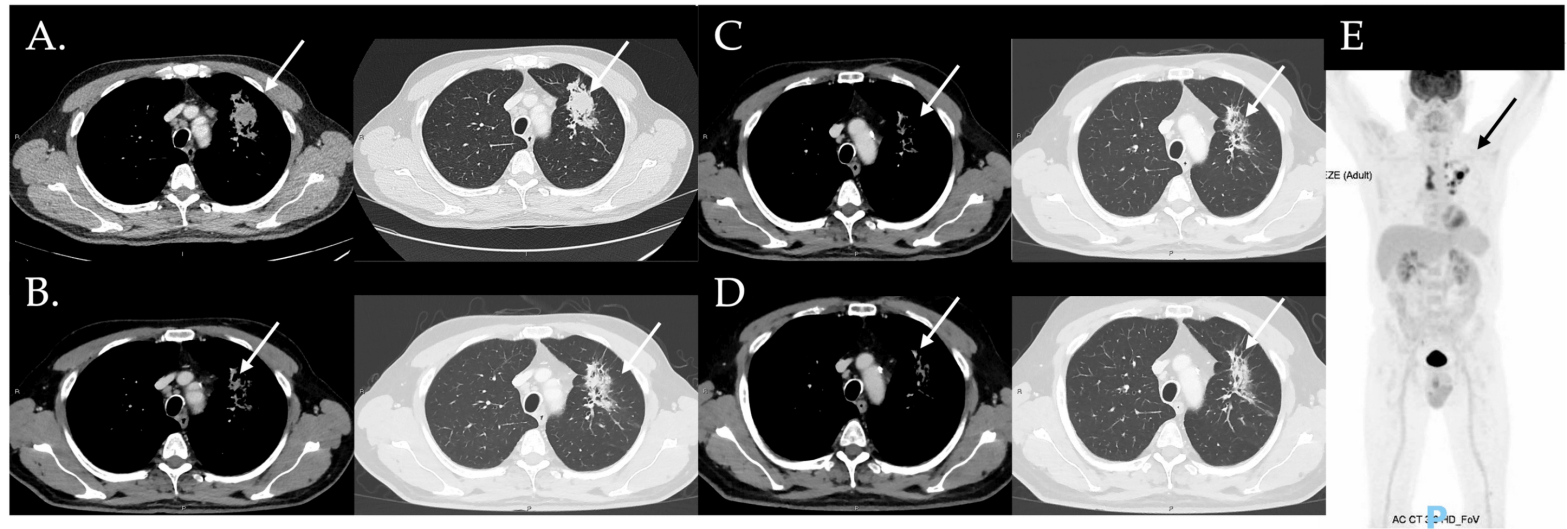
Imaging at diagnosis and follow-up Panels A-D: Thoracic CT scan performed at diagnosis and at six, eight, and 20 months of follow-up, respectively, highlighting the left upper lobe lesion (white arrow). Panel E: Fludeoxyglucose F18 injection PET scan with CT, with no evidence of liver metastasis at the time of cancer diagnosis CT: computed tomography; PET: positron emission tomography
